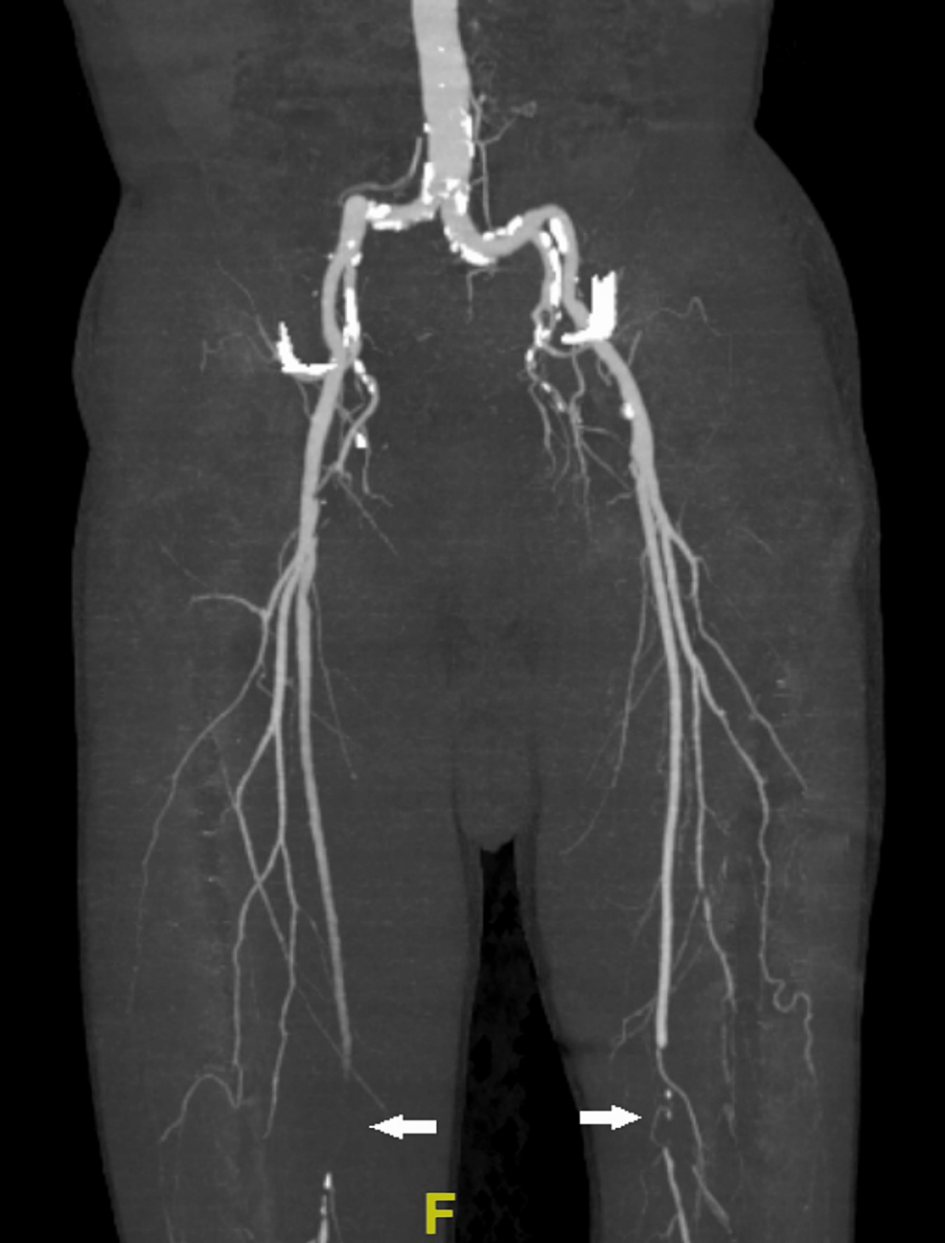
CTA of the abdomen with run-off showed complete occlusion of the right and left superficial femoral arteries (arrows). CTA: computed tomography angiography
